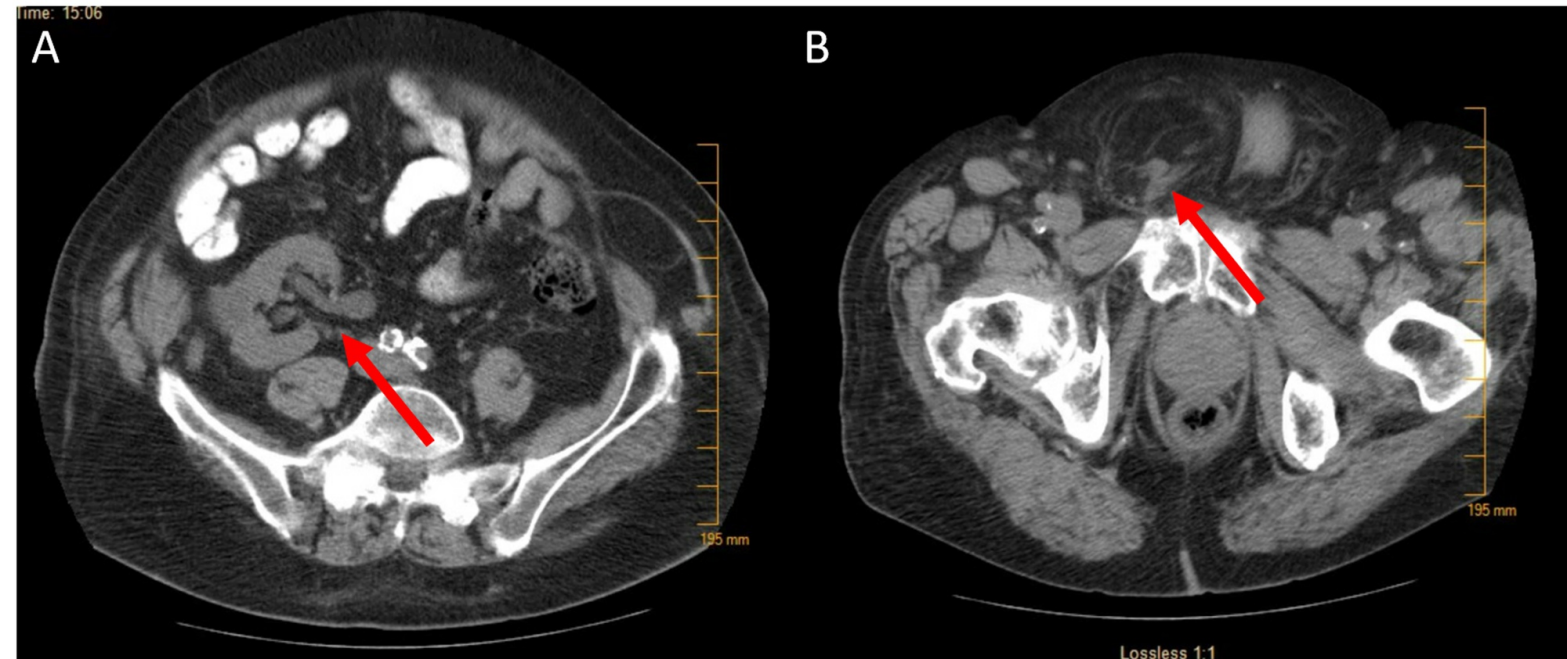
Preoperative CT scan of the abdomen and pelvis A: Right nephroptosis and hydronephrosis (red arrow). B: Right ureter with preperitoneal fat in the scrotum (red arrow).
